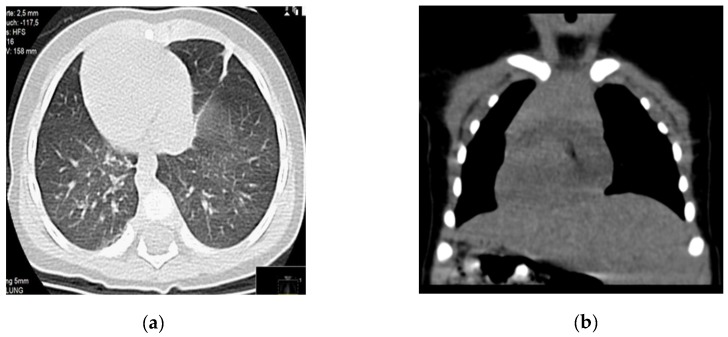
Patient-1 CT scan without contrast. (a) Dextrocardia and normal lung parenchyma. (b) Dextrocardia and left-sided liver.
